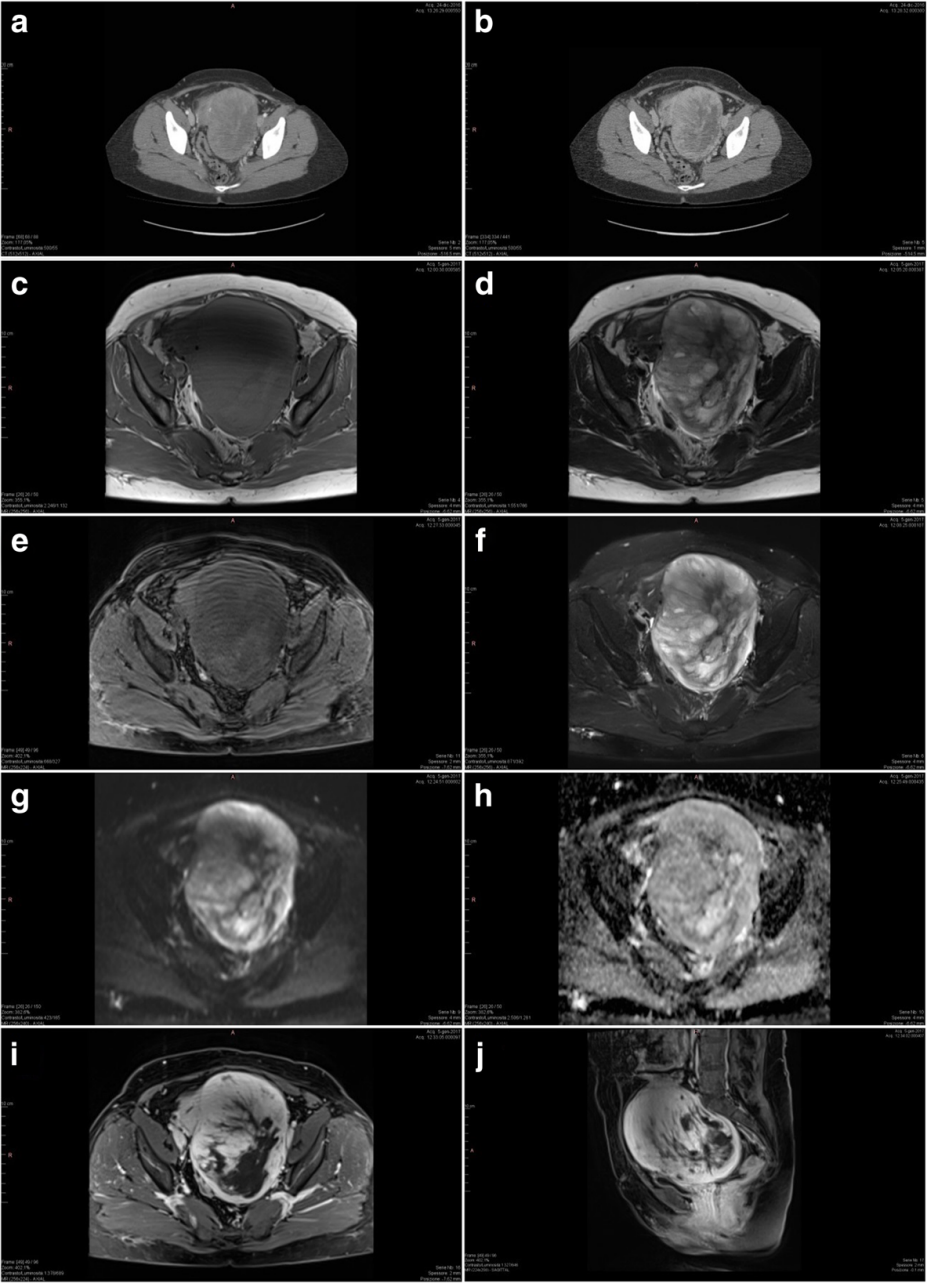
Imaging findings. Computed tomography scan without (a) and with contrast (b) showed the two uterine masses; the large one with eccentric areas of colliquative necrosis. On magnetic resonance imaging, the mass appeared capsulated and not infiltrative. c T1-weighted turbo spin echo (tse), transverse scanning plane (t); d T2-weighted tse; e T1-weighted tse fat suppression (fs), t; f T2-weighted tse fs, t; g Epi spair diffusion-weighted imaging (DWI), t; h Epi spair apparent diffusion coefficient maps (adc), t; i T1 volumetric interpolated breath-hold examination, volumetric interpolated brain examination (vibe) breath holding (bh), t; j T1 vibe bh, sagittal scanning plane (s)
